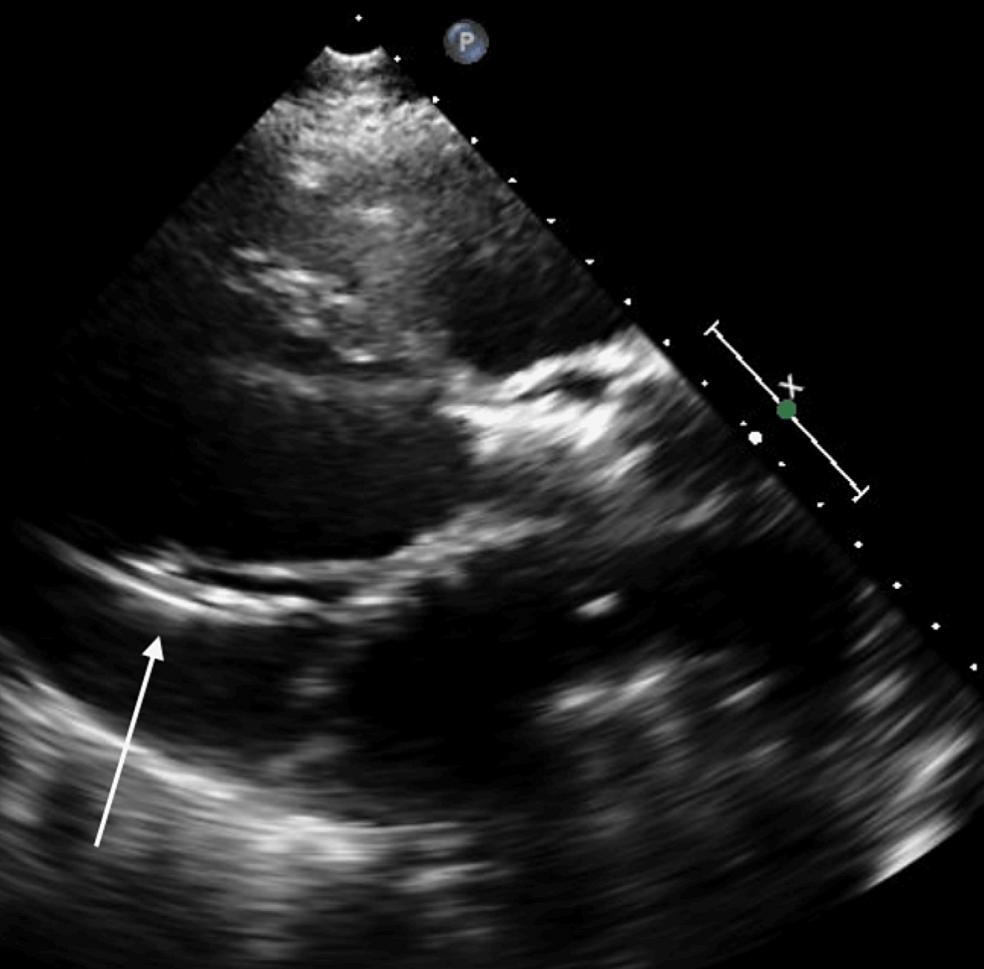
A long parasternal view TTE showing an echo dense mass (white arrow) representing the IABP in the LV TTE: trans-thoracic echocardiogram; IABP: intra-aortic balloon pump; LV: left ventricular
